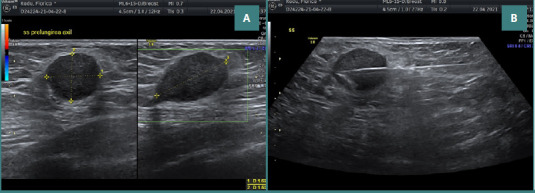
A, The ultrasound characteristics of the lesion revealed a hypoechoic image displaying fine granular content, homogeneity, and a posterior enhancement phenomenon. The contour is slightly irregular in localized areas, and no Doppler signal was detected. B, The lesion was classified as BIRADS 4A, requiring an ultrasound-guided biopsy
